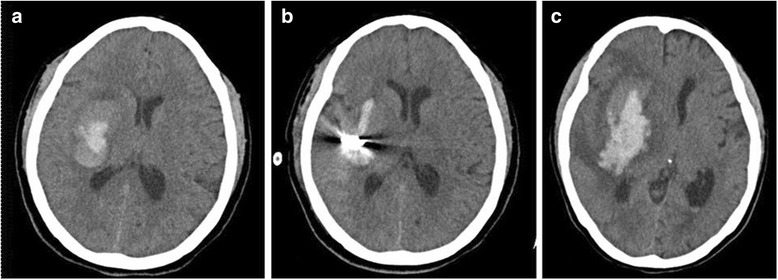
Post-operative re-haemorrhage in patients with blend sign. a The hyper-density on brain CT indicates pre-operative ICH in the right basal ganglion. The hyperdensity is composed of two parts with apparently different CT attenuations. b The ICH was removed with a minimally invasive procedure. c The hyper-density indicates postoperative re-haemorrhage in the same brain area as for the original hematoma
